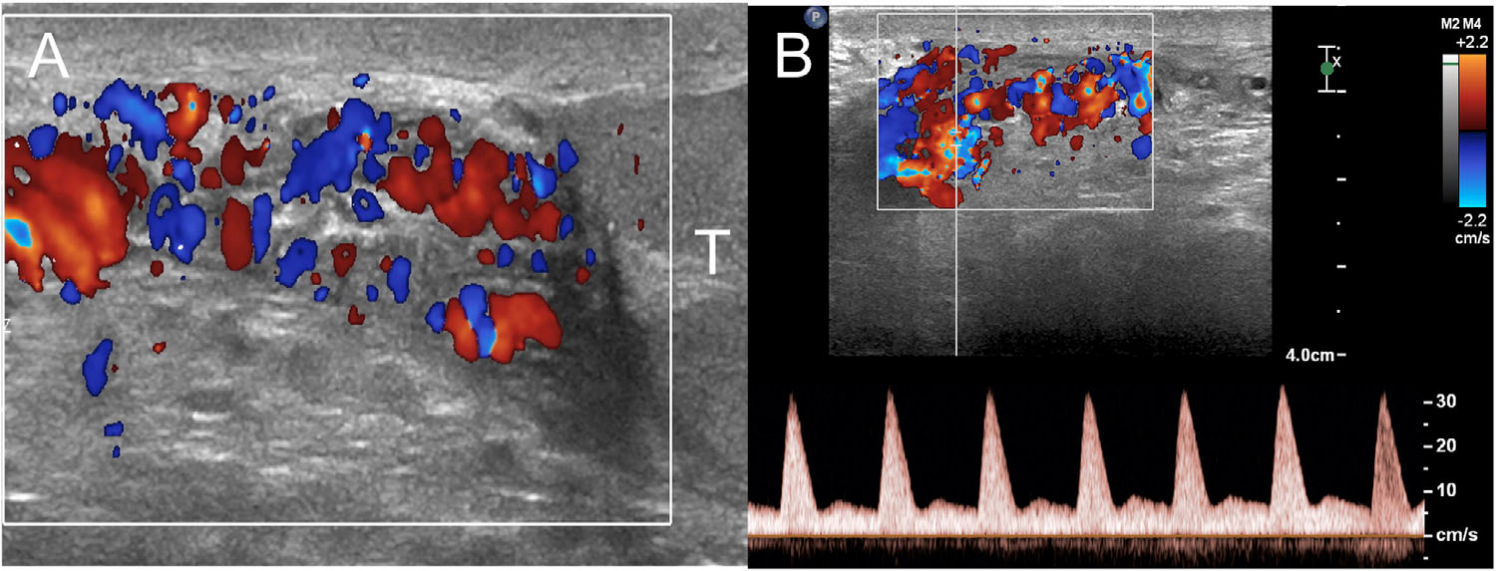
Scotal arteriovenous malformation mimicking varicocoele. (A) Colour Doppler US shows dilated vessels above the testis, resembling supratesticular varicocoele. (B) Spectral Doppler interrogation reveals high velocity arterial flows. (T = testis)
